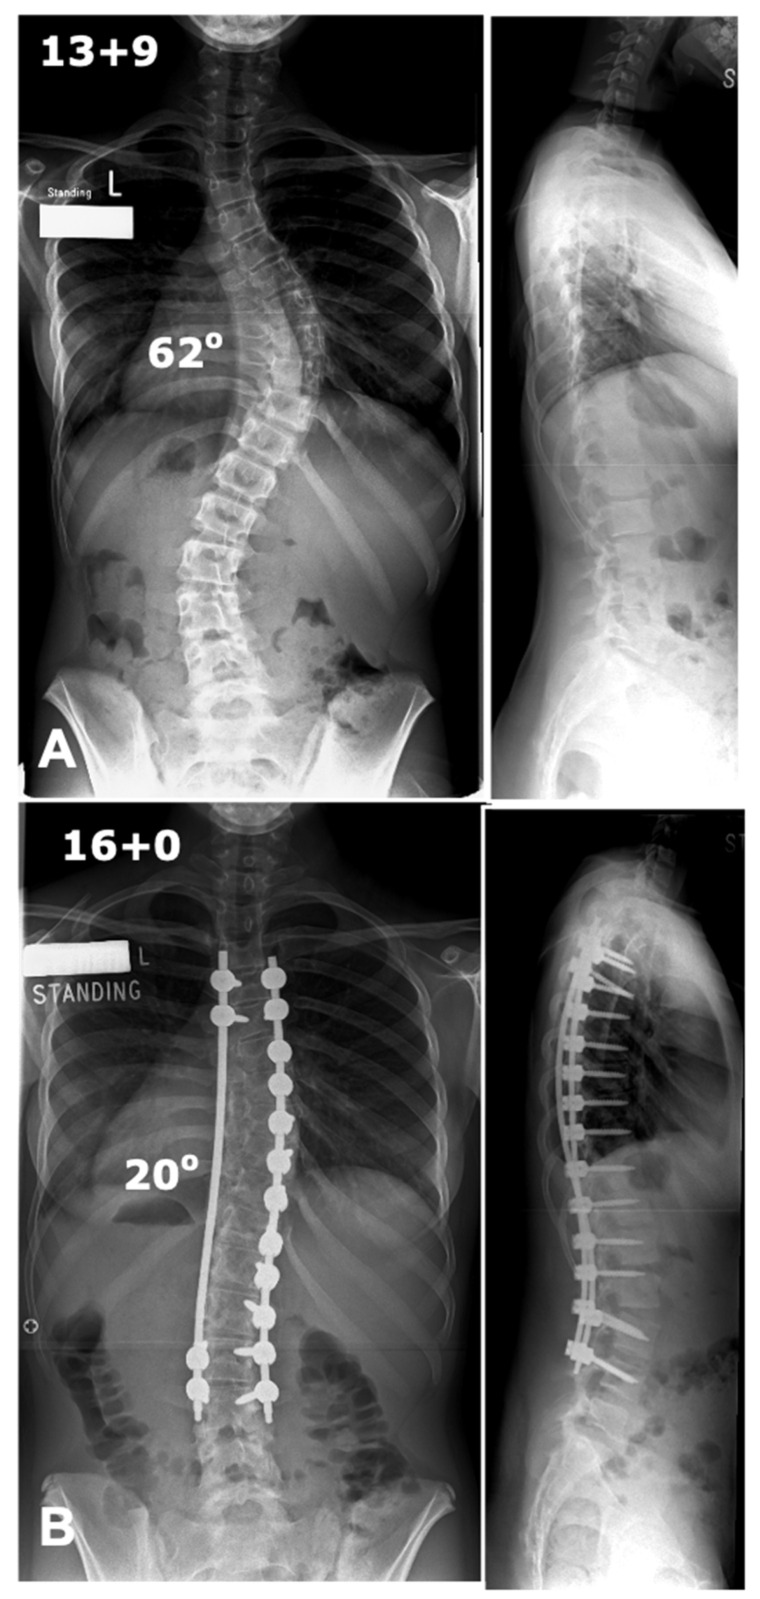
Deformity correction using convex rod technique. (A) Preoperative antero-posterior and lateral radiographs showing a thoracic scoliosis with a Cobb angle of 62 degrees. (B) Postoperative radiographs showing good deformity correction with coronal and sagittal balance.
